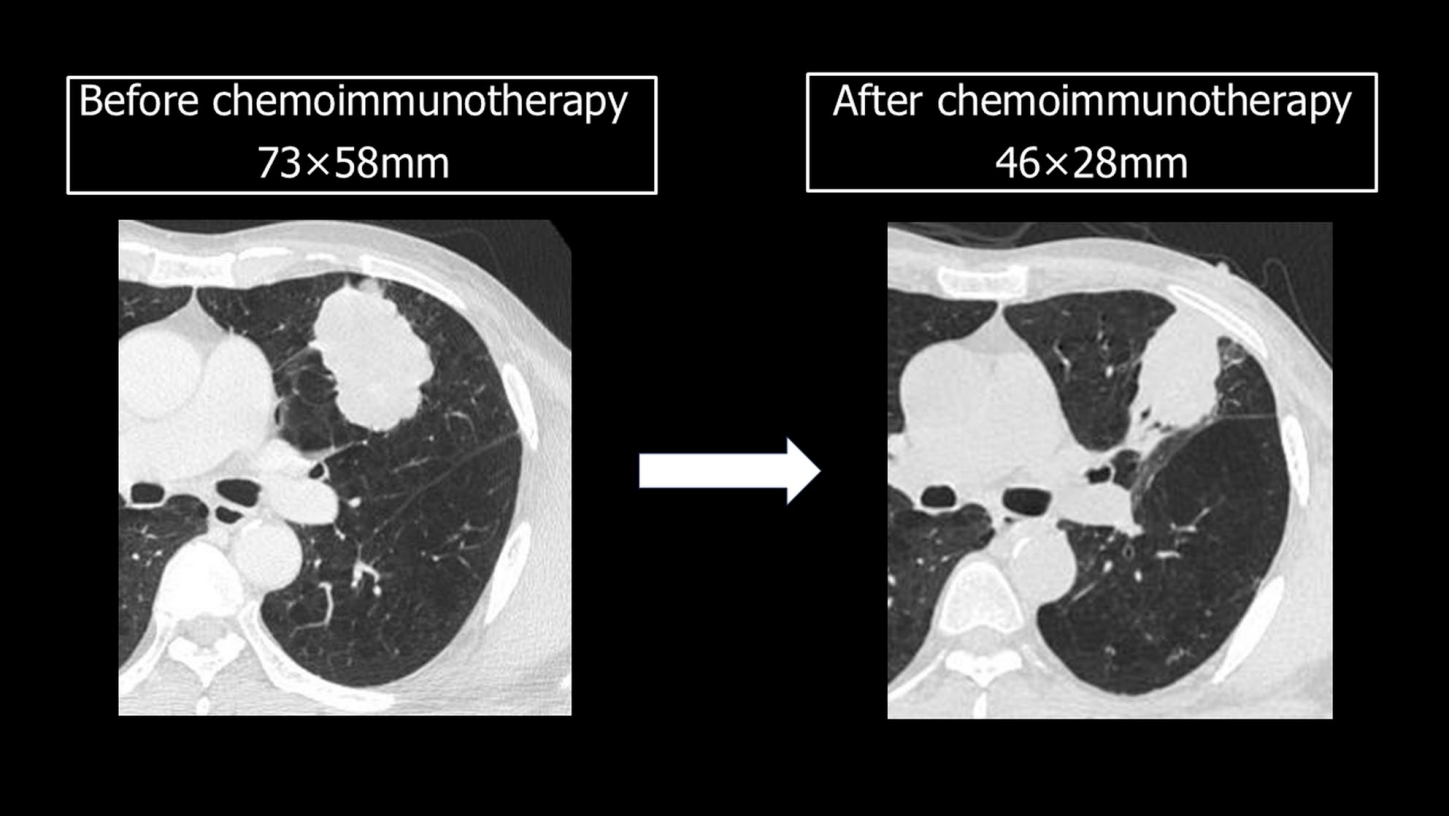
Tumor size before and after neoadjuvant chemotherapy. Chest computed tomography imaging showing the size of the tumor before and after neoadjuvant chemoimmunotherapy and immunotherapy. A 73 × 58-mm mass was observed in the left upper lobe, which shrank to 46 × 28 mm after chemotherapy
